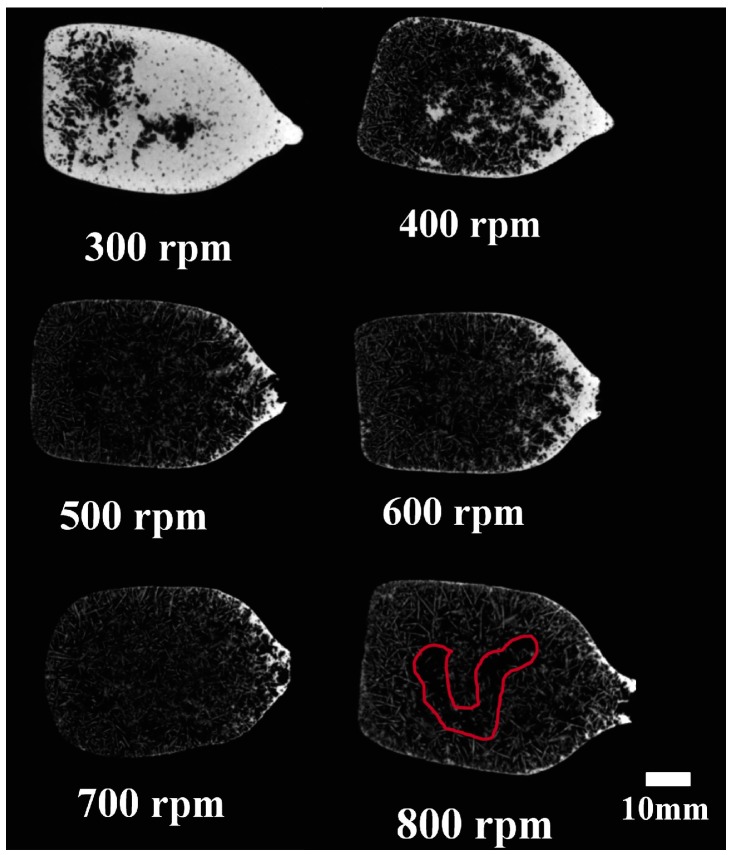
Computed tomography (CT) images of the foams fabricated at various RSs. The area outlined with a red line indicates open structure.
